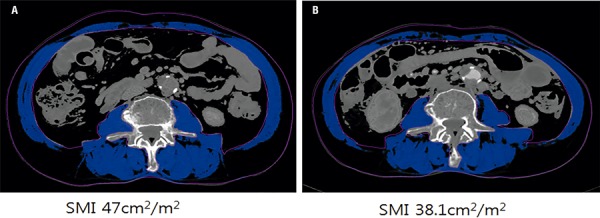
Representative CT scans with decreased skeletal muscle index (SMI) in an 82-year-old man before (A) and at a mean of one year (B) after radical cystectomy.
